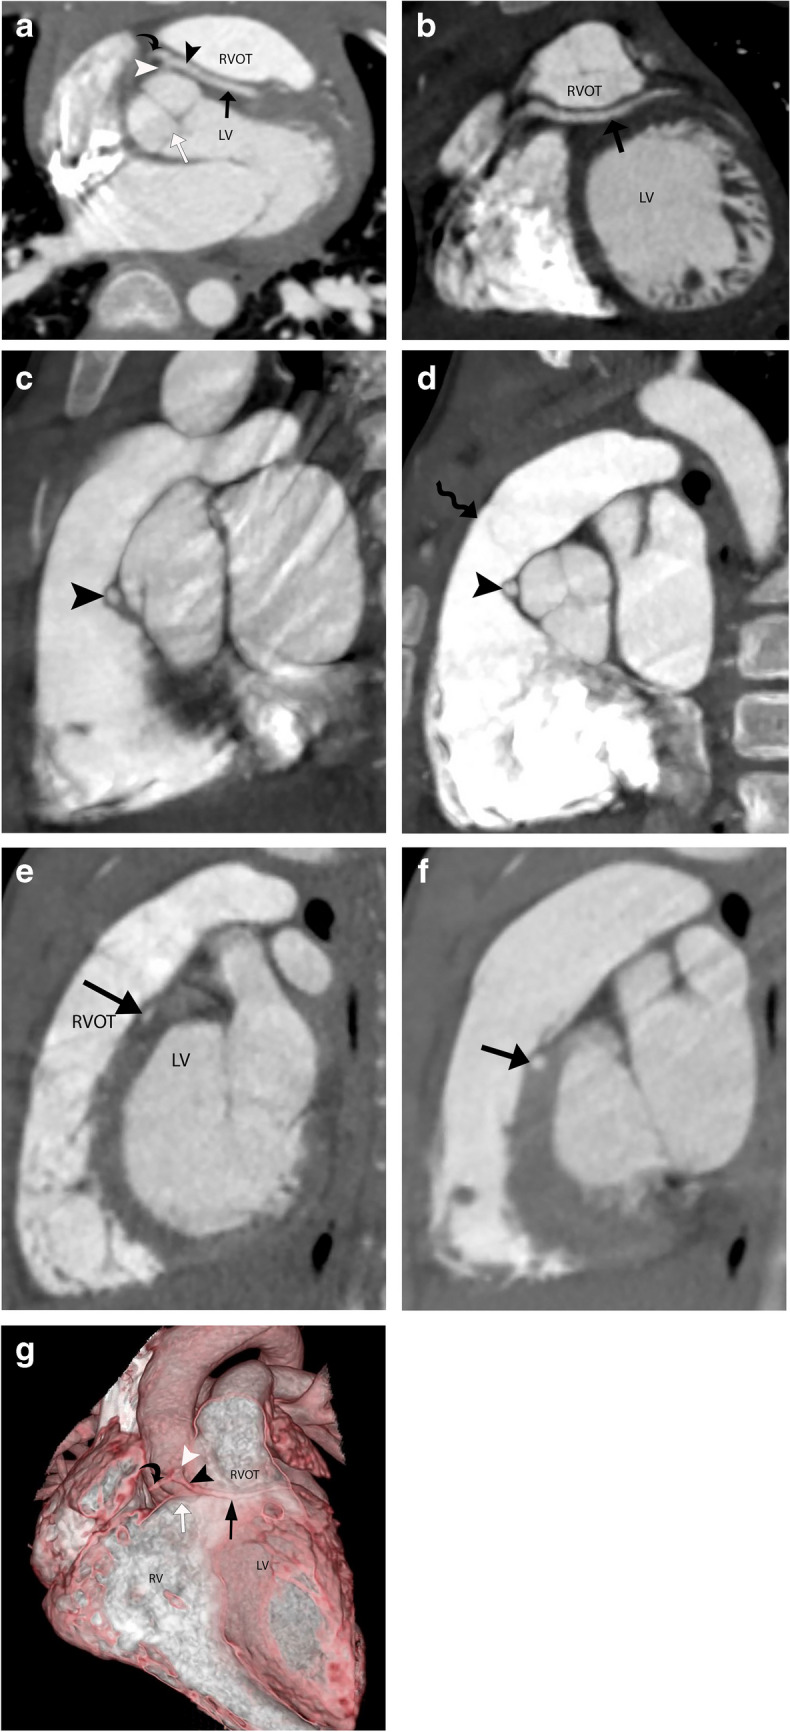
A 4-year-old asymptomatic male with a single coronary artery originating from the right sinus of Valsalva with a transseptal course of the left main coronary artery (LMCA). a Coronary CT angiography (CTA), oblique axial image. A single coronary artery (white arrowhead) originates from the right sinus of Valsalva, which then bifurcates into the right coronary artery (RCA) (black curved arrow) and LMCA (black arrowhead). The LMCA initially courses extraconally (black arrowhead) between the aorta and the right ventricular outflow tract (RVOT). The LMCA dips into the conal septum below the aortic annulus, defining the transseptal segment (black arrow). A white arrow points to the aortic annulus. b Coronary CTA, oblique coronal image. The LMCA (black arrow) courses through the conal septal myocardium with a hammock sign. LV, left ventricle. c Coronary CTA, oblique sagittal image in systolic phase (30% of RR interval). The LMCA (black arrowhead) courses extraconally between the distended aortic root and the contracted RVOT, maintaining a round caliber. d Coronary CTA, oblique sagittal image in diastolic phase (70% of RR interval). The LMCA (black arrowhead) courses extraconally between the aorta and the RVOT (below the level of pulmonary annulus shown by a wavy black arrow). The extraconal segment maintains a round caliber. e Coronary CTA oblique sagittal image in systolic phase (30% of RR interval). The LMCA (black arrow) courses through the septal myocardium between the RVOT and left ventricle (LV). The transconal LMCA (black arrow) shows an elliptical luminal caliber in the systolic phase, suggesting its myocardial compression in the systolic phase, unlike its extraconal segment. f Coronary CTA, oblique sagittal image in diastolic phase (70% of RR interval). The LMCA (black arrow) courses through the conal septal myocardium with a round luminal caliber. g 3D reformatted coronary CTA image, open view through an anterior approach. The LMCA (black arrowhead) originates from a single coronary artery (white arrowhead) arising from the right sinus of Valsalva. The extraconal segment of the LMCA (black arrowhead) extends from its origin to the level of the aortic annulus (white arrow ) between the aorta and RVOT. The intraconal segment is the hammock-like transverse segment (black arrow) below the level of the aortic annulus through the posterior wall of RVOT
